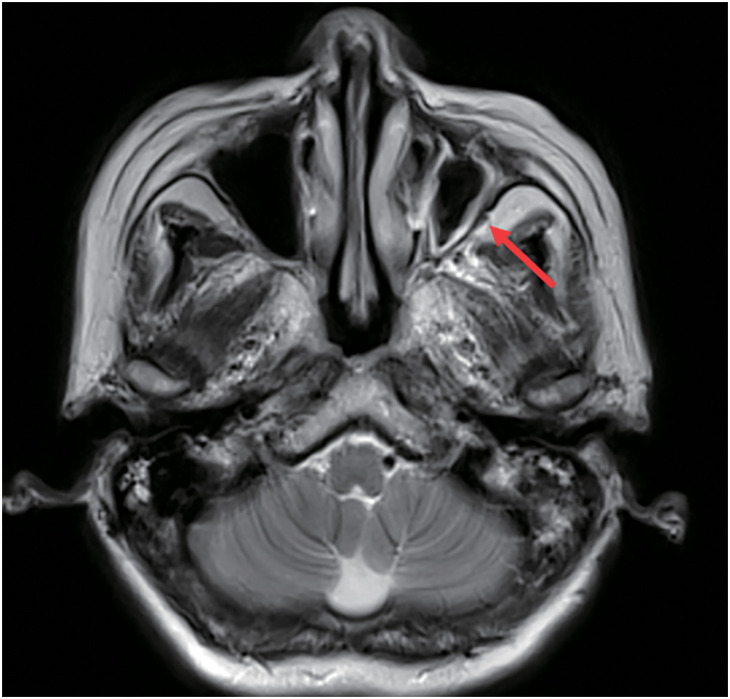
Cranial magnetic resonance imaging. Cranial magnetic resonance imaging demonstrated the presence of sinusitis (the red arrow) in the patient.
